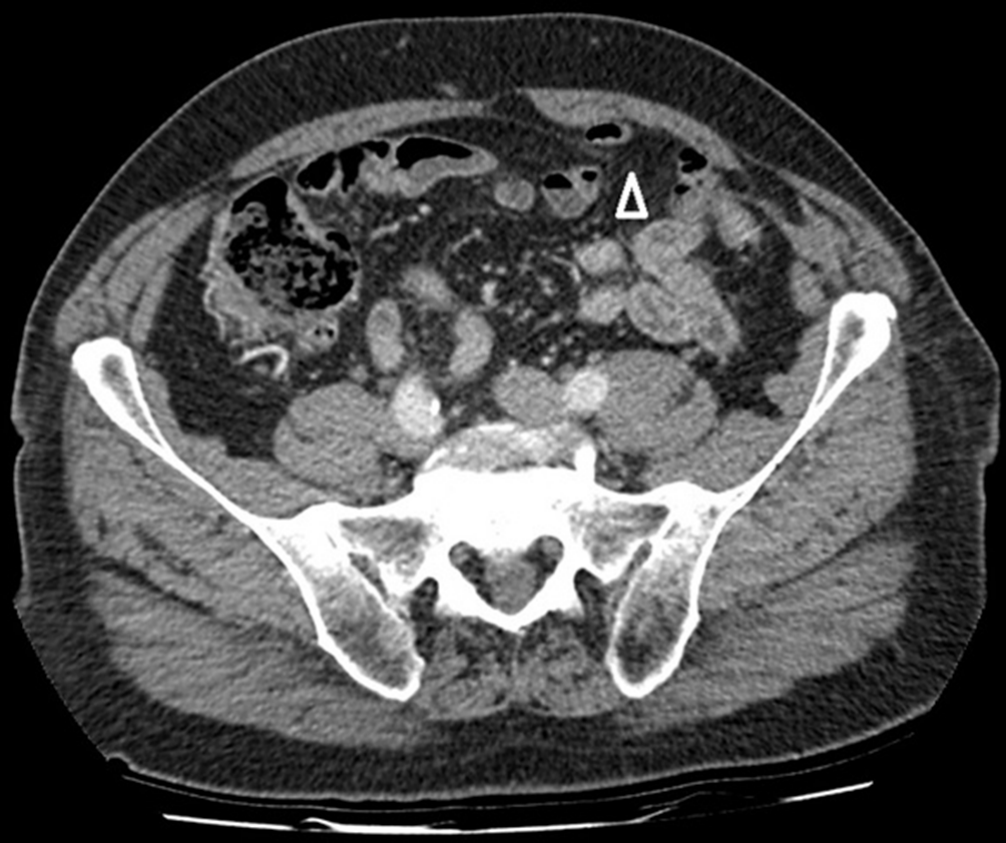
A contrast-enhanced, portal venous phase axial CT image at a lower level reveals the defect (arrowhead) which is located in the infraumbilical region, below the arcuate line.
